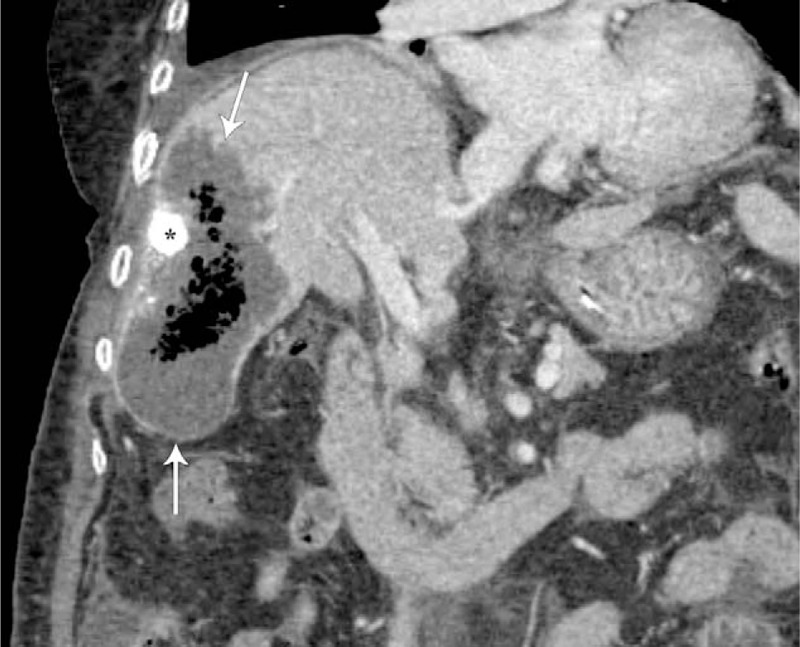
Five-week follow-up contrast-enhanced coronal CT image shows a more aggravated air-filled abscess (arrows) around a compact lipiodolized lesion (asterisk) in the right hepatic lobe, despite percutaneous drainage. CT = computed tomography.
